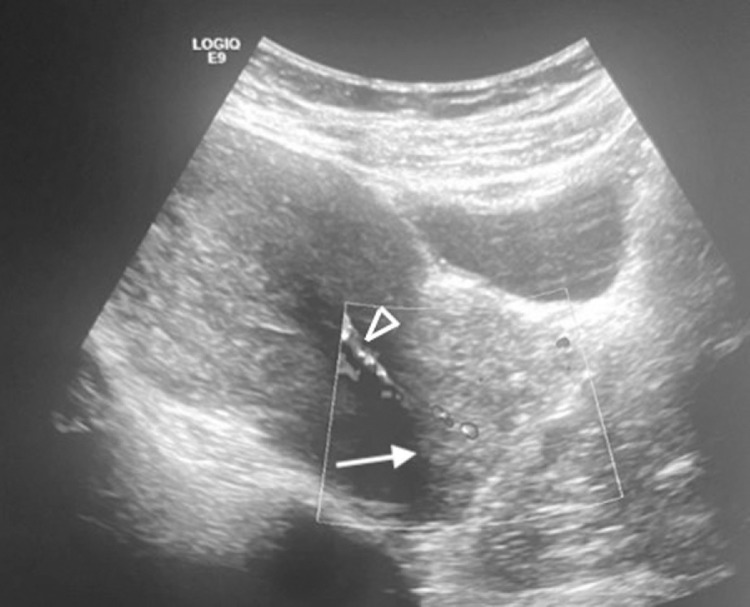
Median sagittal view of the suprapubic pelvic ultrasonography done on our patient, showing a cervical mass of heterogenous echopattern (white arrow), with visualization of the feeding artery in Doppler mode (arrowhead).
